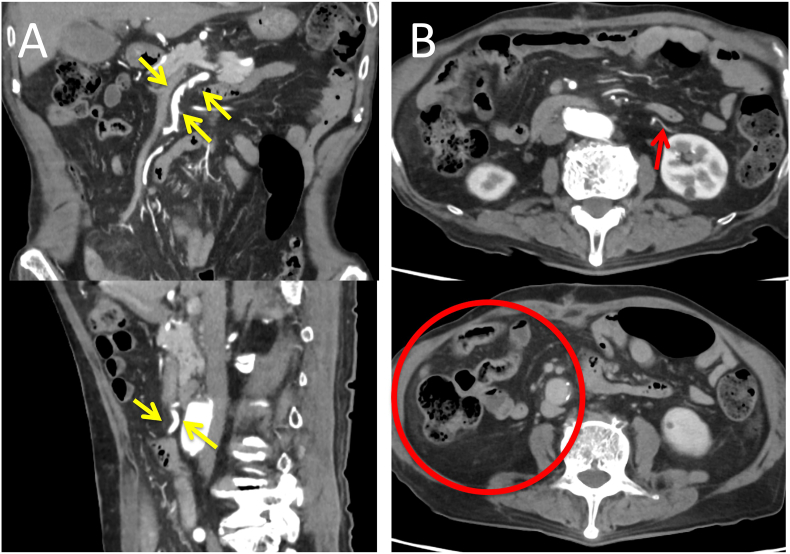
Contrast-enhanced CT on day 7 after anticoagulation A: Image showing resolution of the SMA thrombus. B: Contrast enhancement improved despite edematous changes in mesentery.
